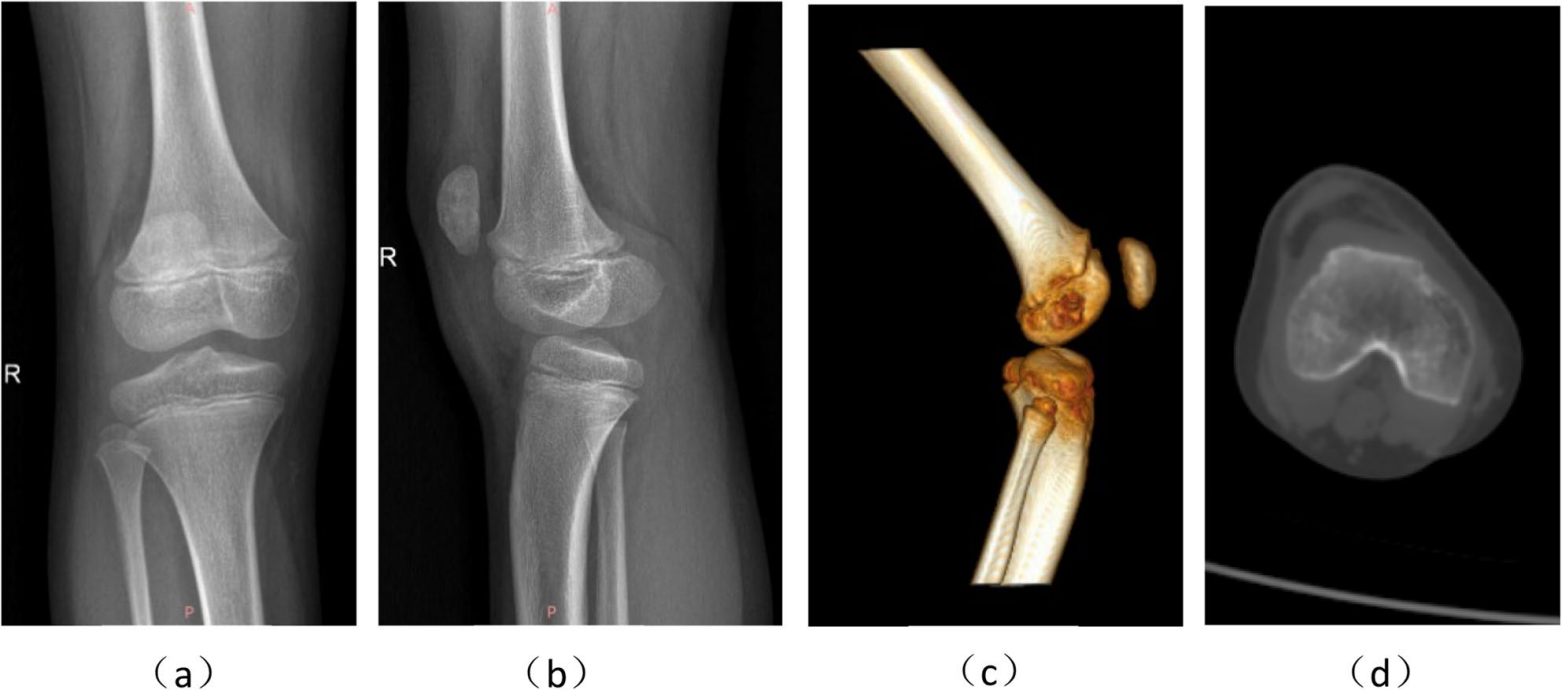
Preoperative radiographs and computed tomography (CT) images of the knee joint. a The anteroposterior radiograph of the right knee joint shows a visible, unclosed epiphysis and subluxated patella; (b) lateral radiograph of the right knee joint demonstrating patella alta; c CT three-dimensional CT reconstruction of the right knee joint demonstrating patella alta; d horizontal CT of the right knee joint showing a low, flat femoral trochlea and the Dejour type D trochlea
